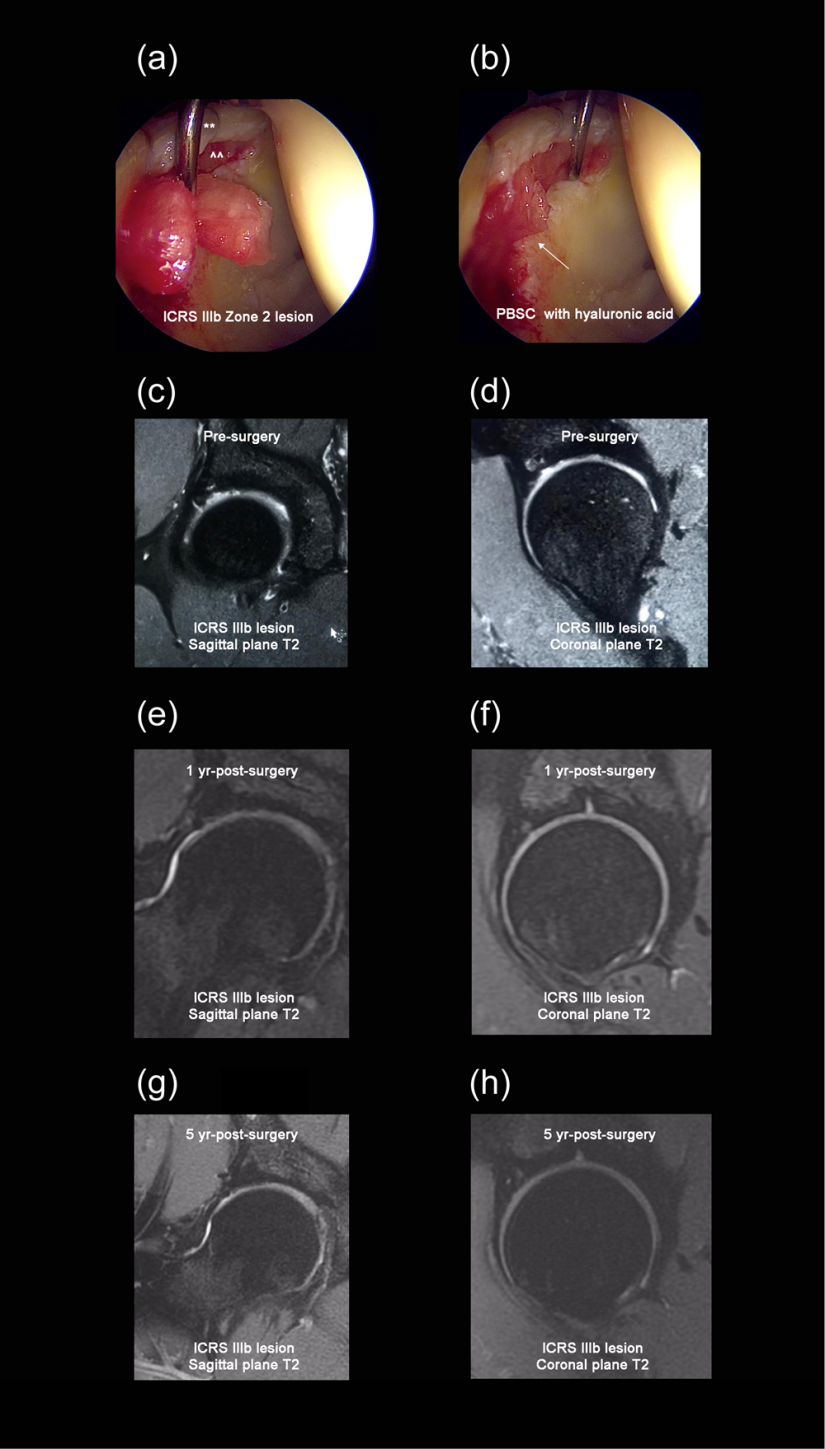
(a) 34‐year‐old male with International Cartilage Repair Society (ICRS) IIIb Zone 2 osteochondral lesion during the placement of the infiltration of peripheral blood stem cells (PBSC) with hyaluronic acid.  = repaired labrum. ^^ = chondral lesion. (b) Final placement of the PBSC with hyaluronic acid (white arrow) in the chondral lesion for the same 34‐year‐old male patient. (c and d) A T2 sagittal and coronal MRI of a 24‐year‐old male with ICRS IIIb lesion. (e and f) A T2 sagittal and coronal MRI of the same 24‐year‐old male patient at 1 year post‐surgery. (g and h) A T2 sagittal and coronal MRI of the same 24‐year‐old male patient at 5 years postsurgery showed a complete cartilage lesion repaired, stable, and complete subchondral bone repaired.
